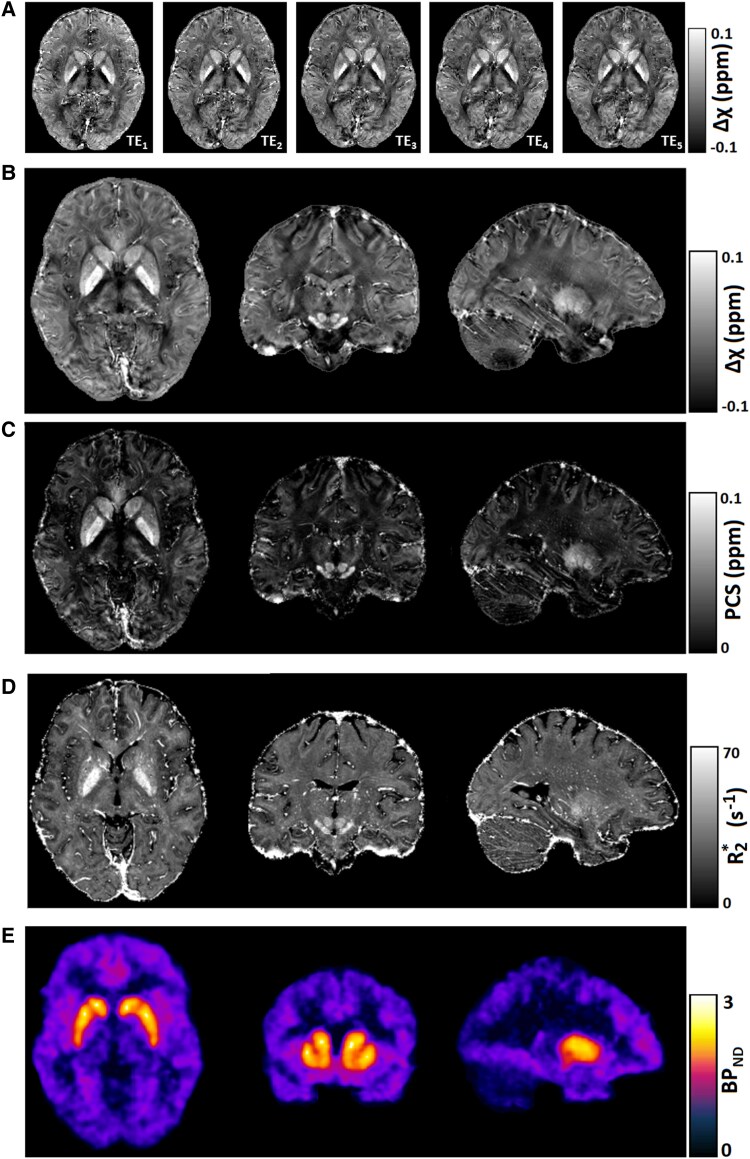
Examples of susceptibility-sensitive MRI and D_1_ receptor availability PET maps obtained in a randomly selected subject. (A) QSM estimates of the first five echoes acquired at echo times $TE_{1}$, …, $TE_{5}$, which were subsequently combined for the final QSM reconstruction of Δχ. (B) Axial, coronal and sagittal planes of the final QSM result. (C) Corresponding PCS results obtained with DECOMPOSE. (D) Corresponding $R_{2}^{*}$ maps. (E) Example of a [^11^C]SCH23390 $BP_{\mathrm{ND}}$ map.
